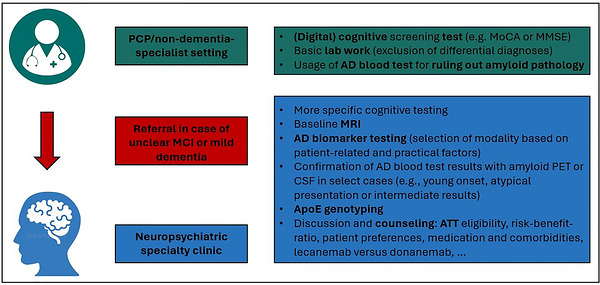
Patient flow along the AD diagnostic process and determination of ATT eligibility. AD, Alzheimer's disease; *APOE*, apolipoprotein E; ATT, amyloid‐targeting treatment; CSF, cerebrospinal fluid; MCI, mild cognitive impairment; MMSE, Mini‐Mental State Examination; MoCA, Montreal Cognitive Assessment; MRI, magnetic resonance imaging; PET, positron emission tomography; PCP, primary care physician.
